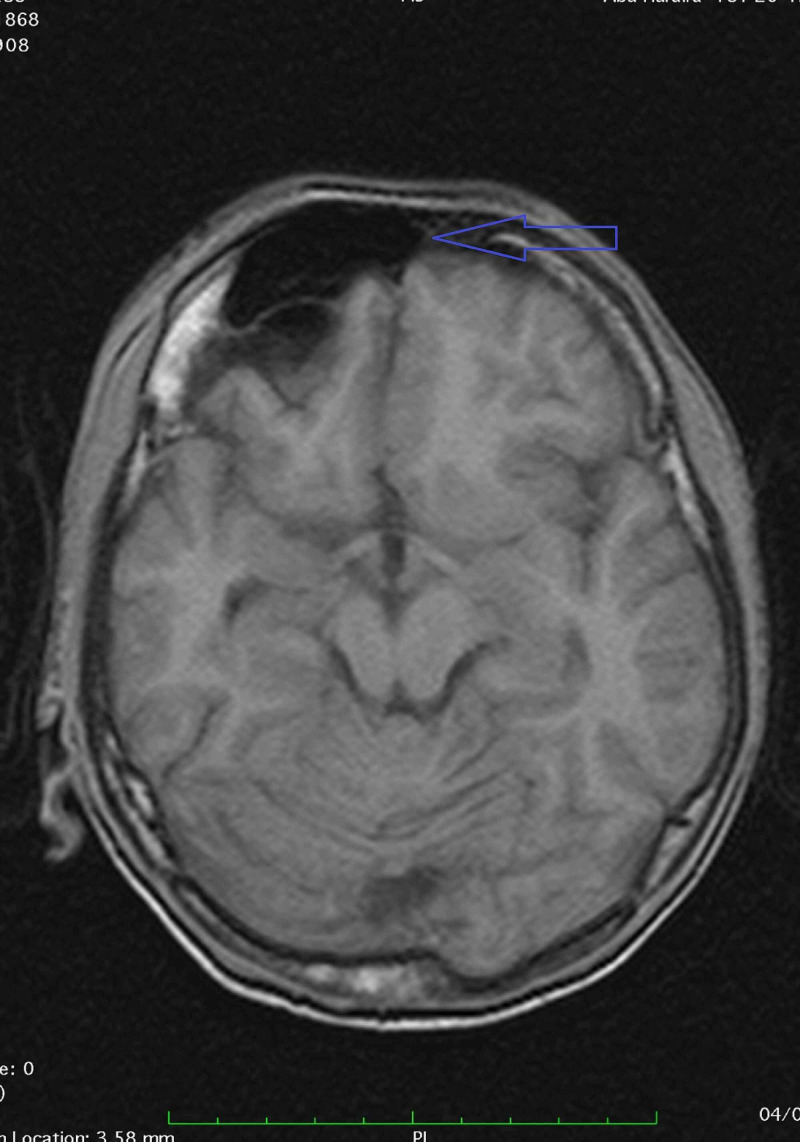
MRI showing hyperpneumatization of right frontal sinus (blue arrow) as compared to the left one
